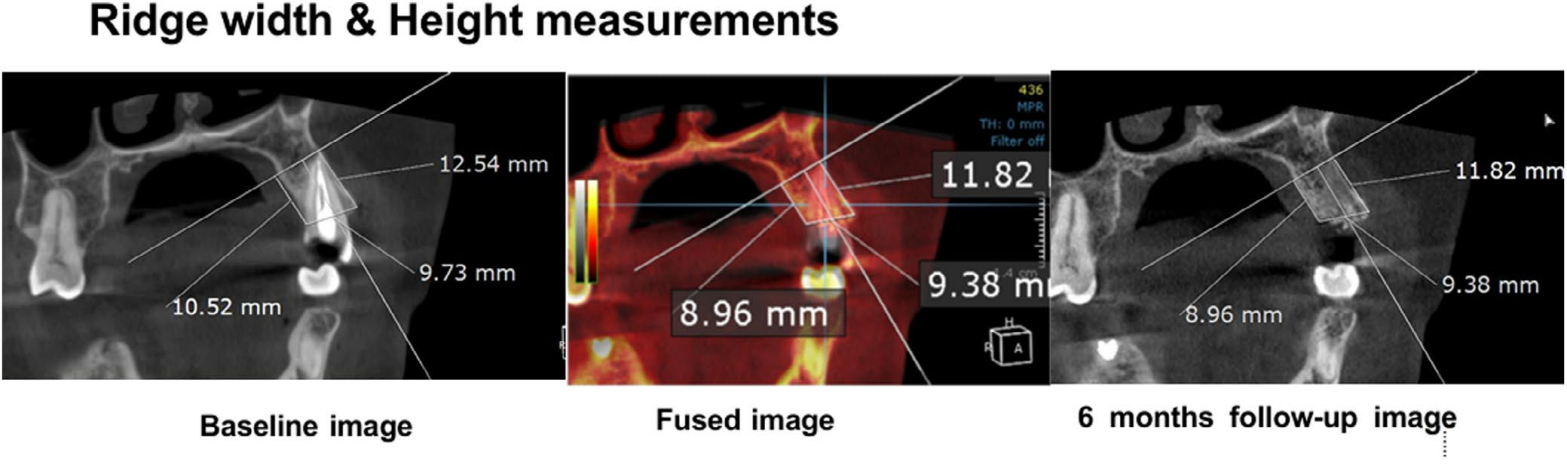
Radiographic examination, a CBCT at baseline (left) and 6 months postoperatively (right) with the fused radiographic cross‐section cut (middle).
